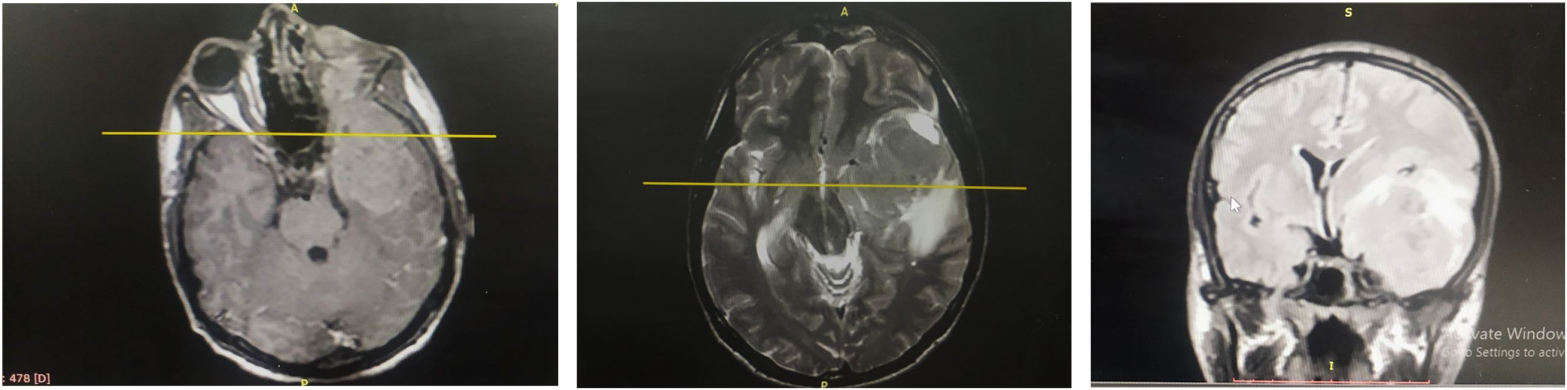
Brain MRI (T1W, T2W, and FLAIR): A heterogeneous mass measuring 9 × 7.5 × 3 cm in the left temporal region, extending to the wing of the sphenoid and causing destruction of the lateral wall of the pilgrims, compressing the middle cerebral artery and infiltrating in the cavernous sinus.
